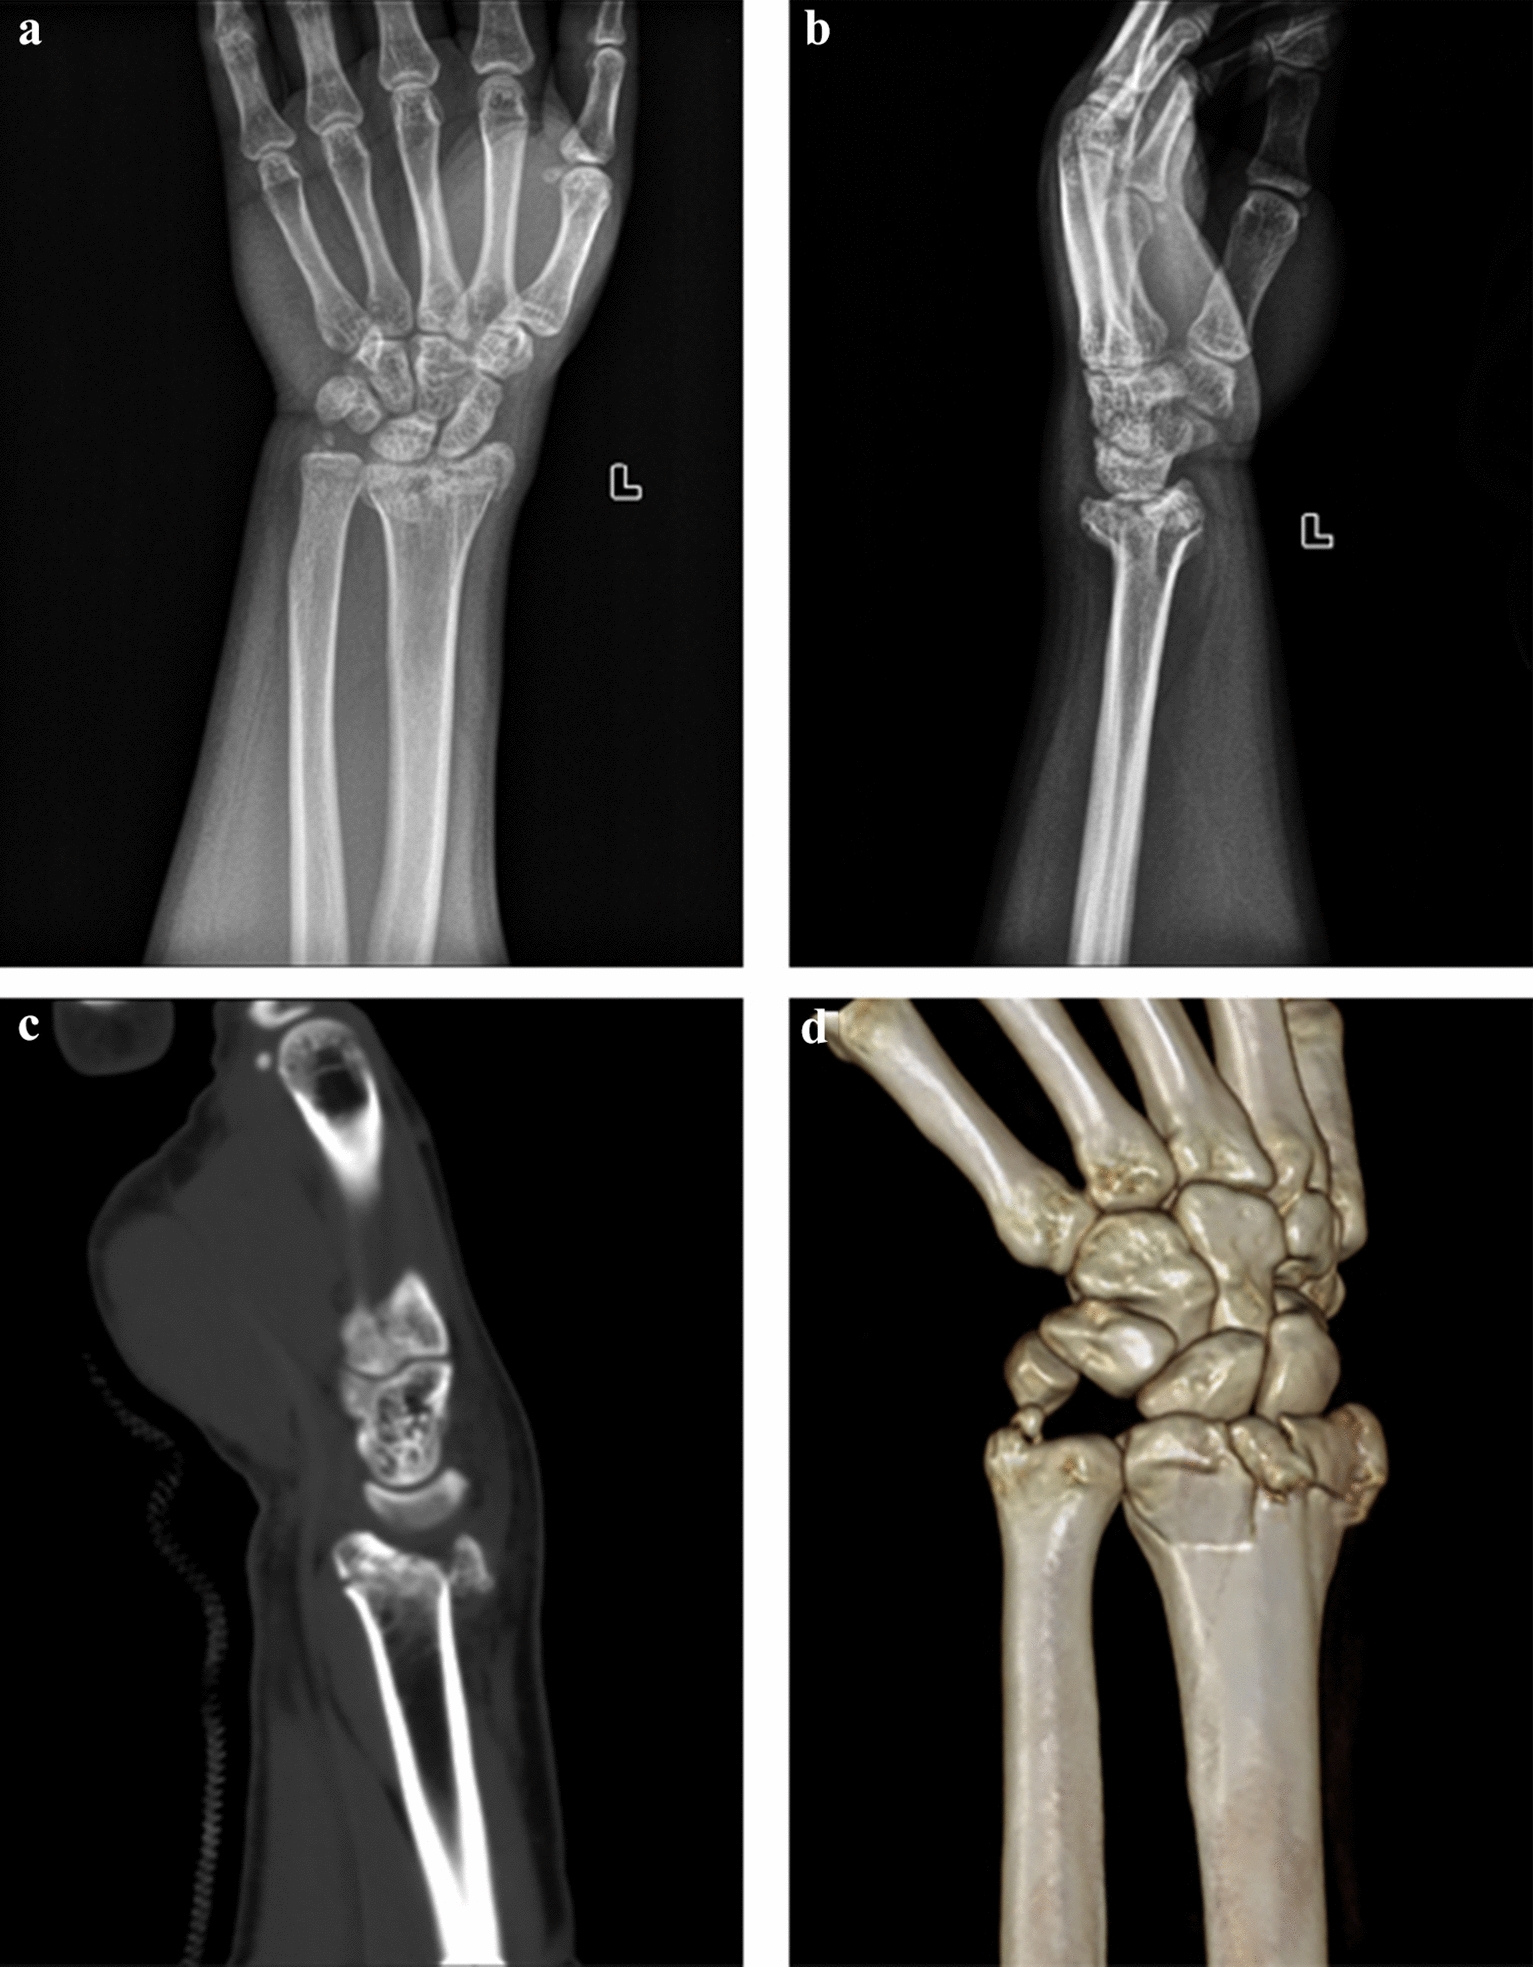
A 42-year-old man suffered from a comminuted fracture of the left distal radius after falling from a high place. A dorsal free fracture fragment is shown at the distal radius. a, b Anteroposterior and lateral X-rays before surgery. c, d Computed tomography of the fracture
